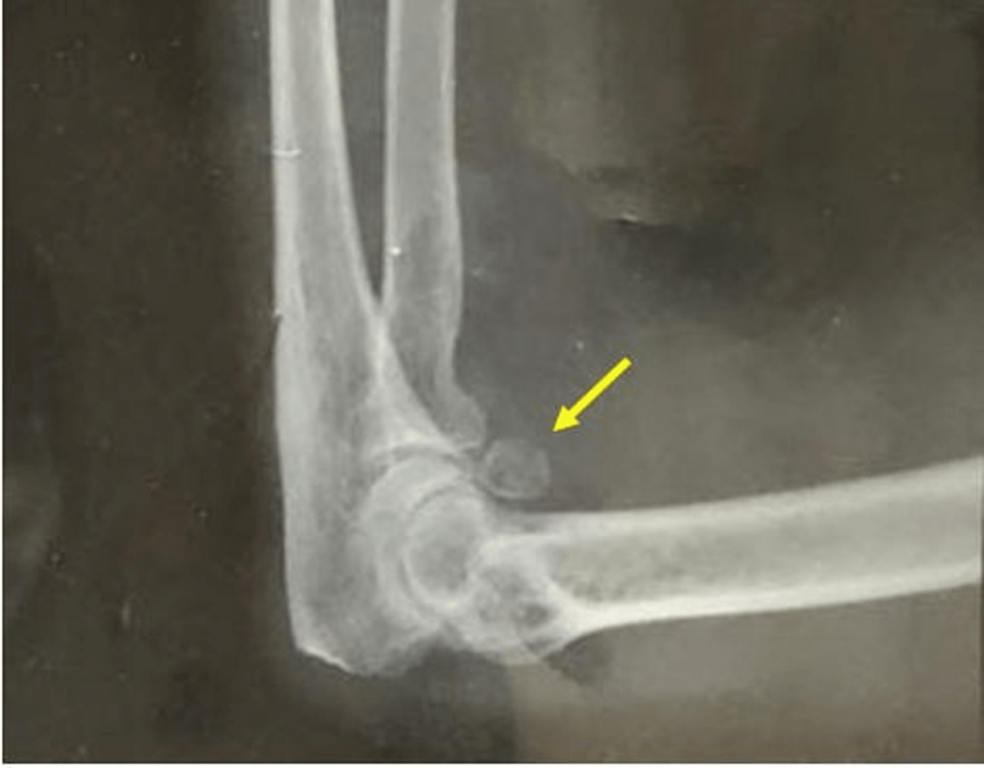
X-ray left elbow joint lateral view shows osteoporotic changes in the proximal radius, ulna, and distal humerus with narrowing of joint space and an intra-articular loose body (arrow)
